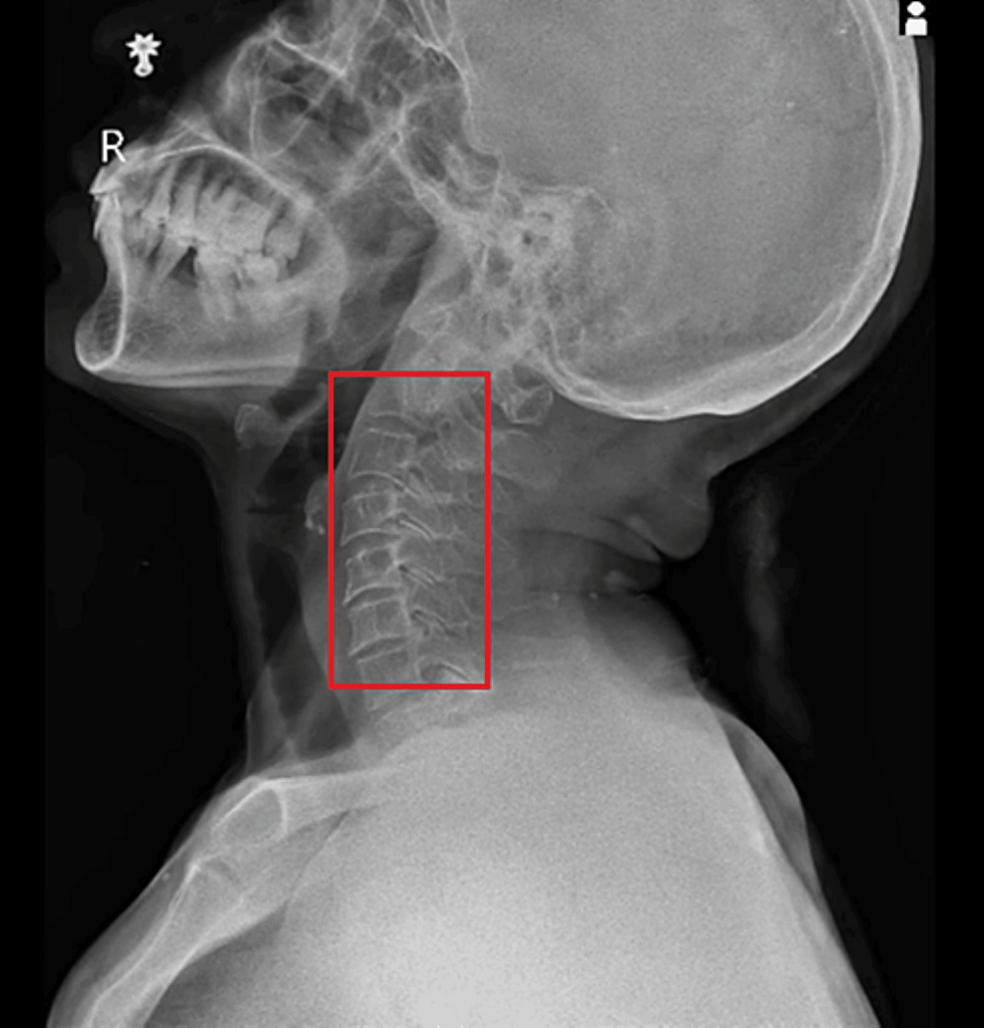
X-ray of cervical spondylosis
